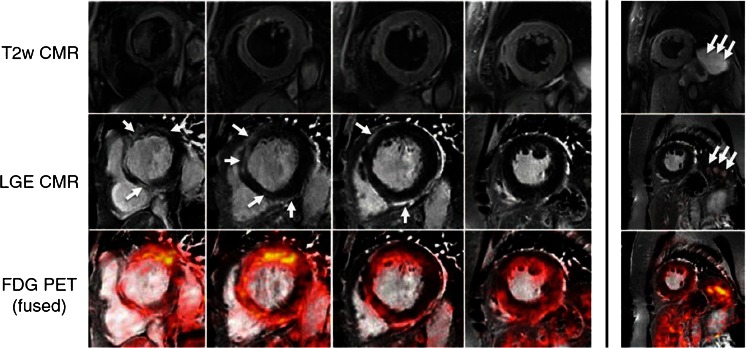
A 72-year-old woman was referred with increasing shortness of breath and intermittent chest pain. Echocardiography revealed a diminished left ventricular ejection fraction (35 %); significant CAD could be ruled out by coronary angiography. Biopsy of an enlarged scalene lymph node showed non-caseating granulomas, so cardiac sarcoid was considered, and a hybrid PET/MRI was scheduled. In the *upper row* T2-weighted images are displayed in cine short axis views. *Middle row* shows the concomitant LGE images; the presence of LGE is indicated by the *white arrows*. In the *bottom row*, there are fused images (MRI and PET): note that PET images show an increased uptake in the border zones of the LGE, suggesting that the process of inflammation affects more regions than displayed solely by LGE. *Far right*, CMR images showing splenic nodules with enhancement on both LGE sequences and by PET. *Reprinted with permission from LWW* [42]
